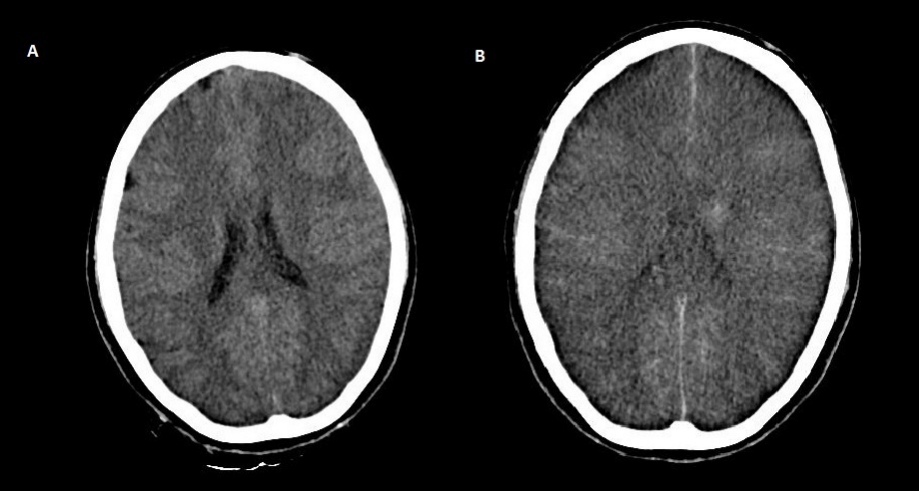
Computed tomography (CT) scans on admission and on the last day of admission Panel A - Normal CT scan on admission. No evidence of cerebral edema or infarction/bleeding. Panel B - Follow-up CT scan showing marked diffuse brain edema with effacement of internal and external cerebrospinal fluid (CSF) spaces suggestive of Global cerebral ischemia.
